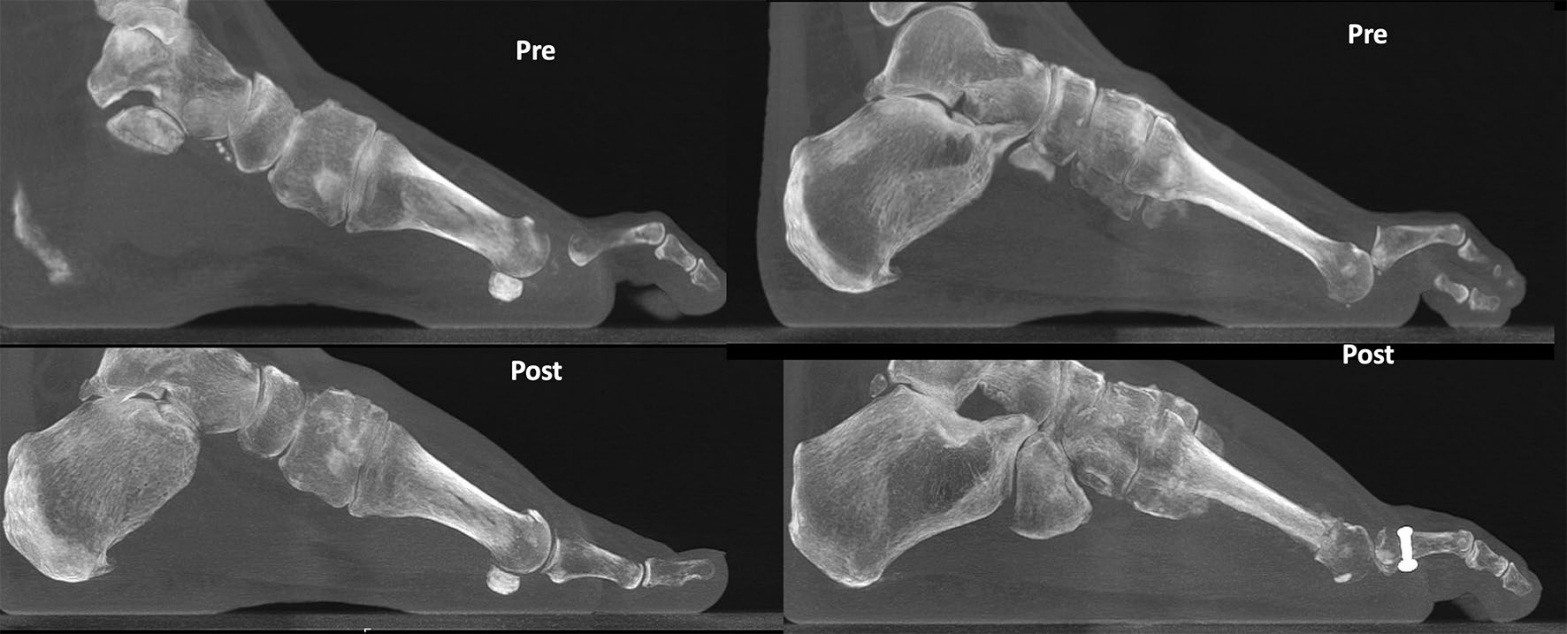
Preoperative and 3-months postoperative wide sagittal weight bearing CT images of patient’s foot 1st and 2nd rays demonstrating preoperative mild collapse of the medial column (Left) as well as lesser 2nd toe deformity and dorsal subluxation of 2nd metatarsophalangeal joint (MTPJ) (Right). Considerable correction of the medial column (Left) and the 2nd toe deformity and MTPJ reduction is postoperatively (Right)
